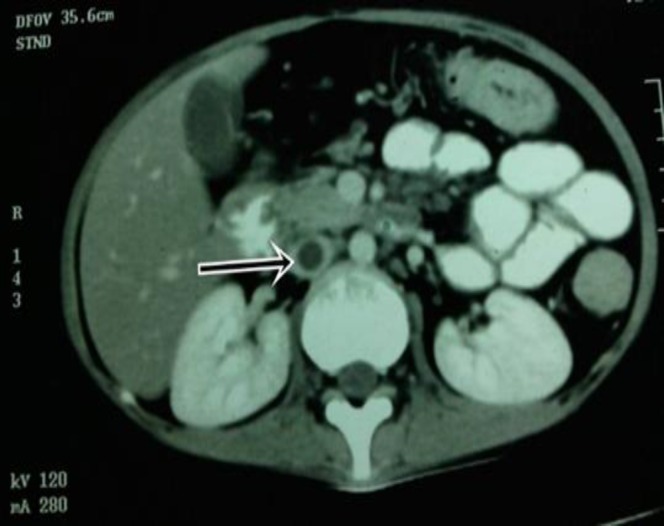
CT abdomen showing thrombus in IVC
